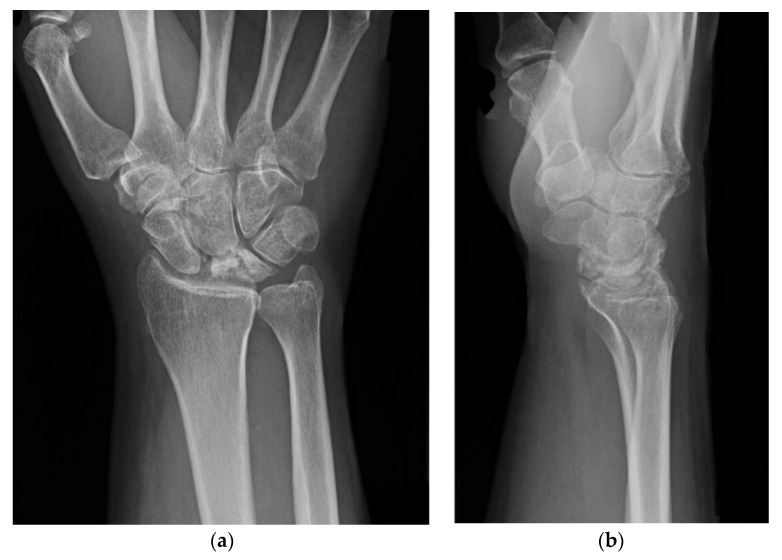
X-ray stage 3B: the collapse of lunate, carpal instability, scaphoid rotation, radioscaphoid angle (RSA) increased. (a) Stage 3B, AP view X-ray; (b) Stage 3B, lateral view -ray.
